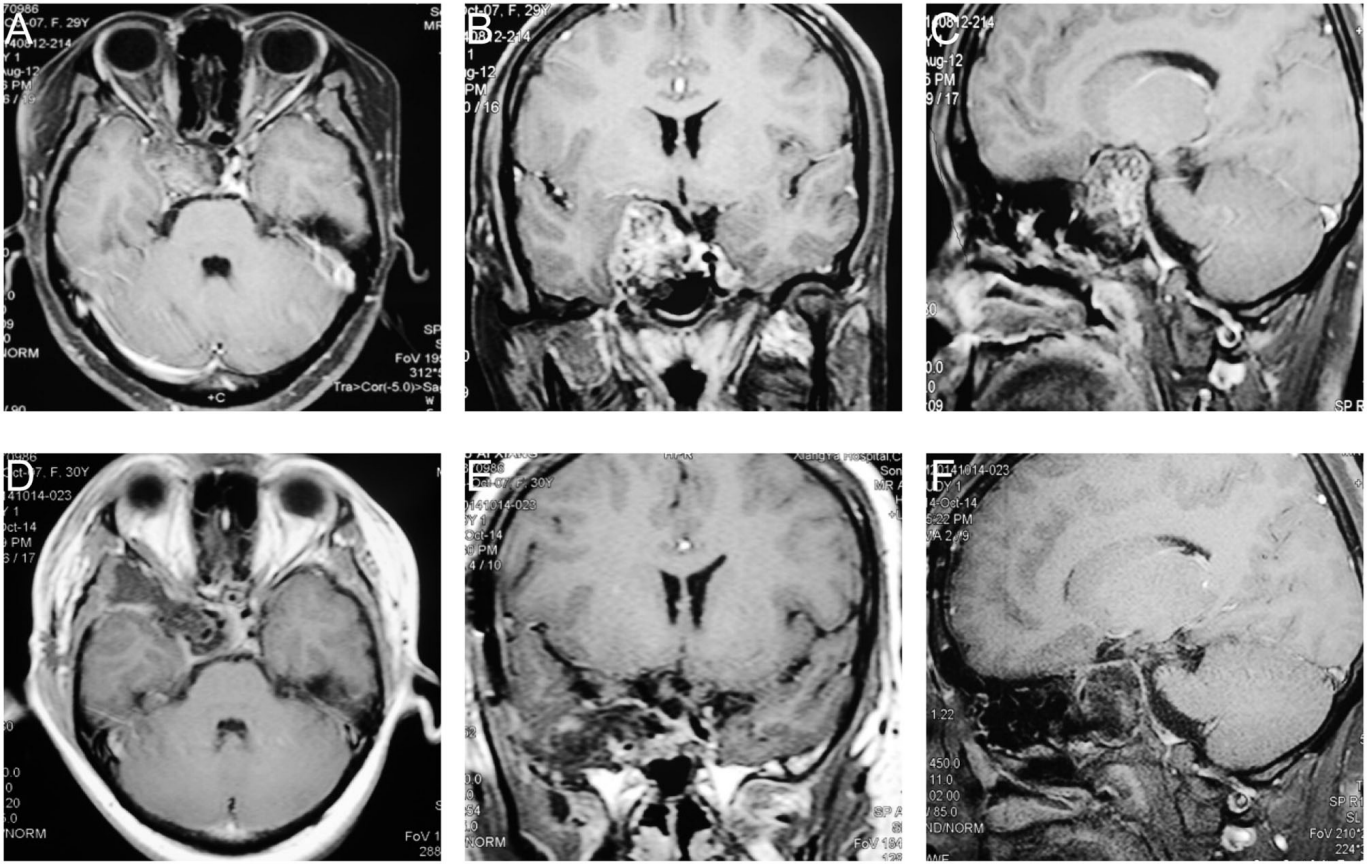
The preoperative and postoperative MRI imaging of case 1. (A–C) Preoperative MRI showed the tumor involved intrasellar and the right CS. (D–F) Postoperative MRI showed the tumor was gross total resected.
